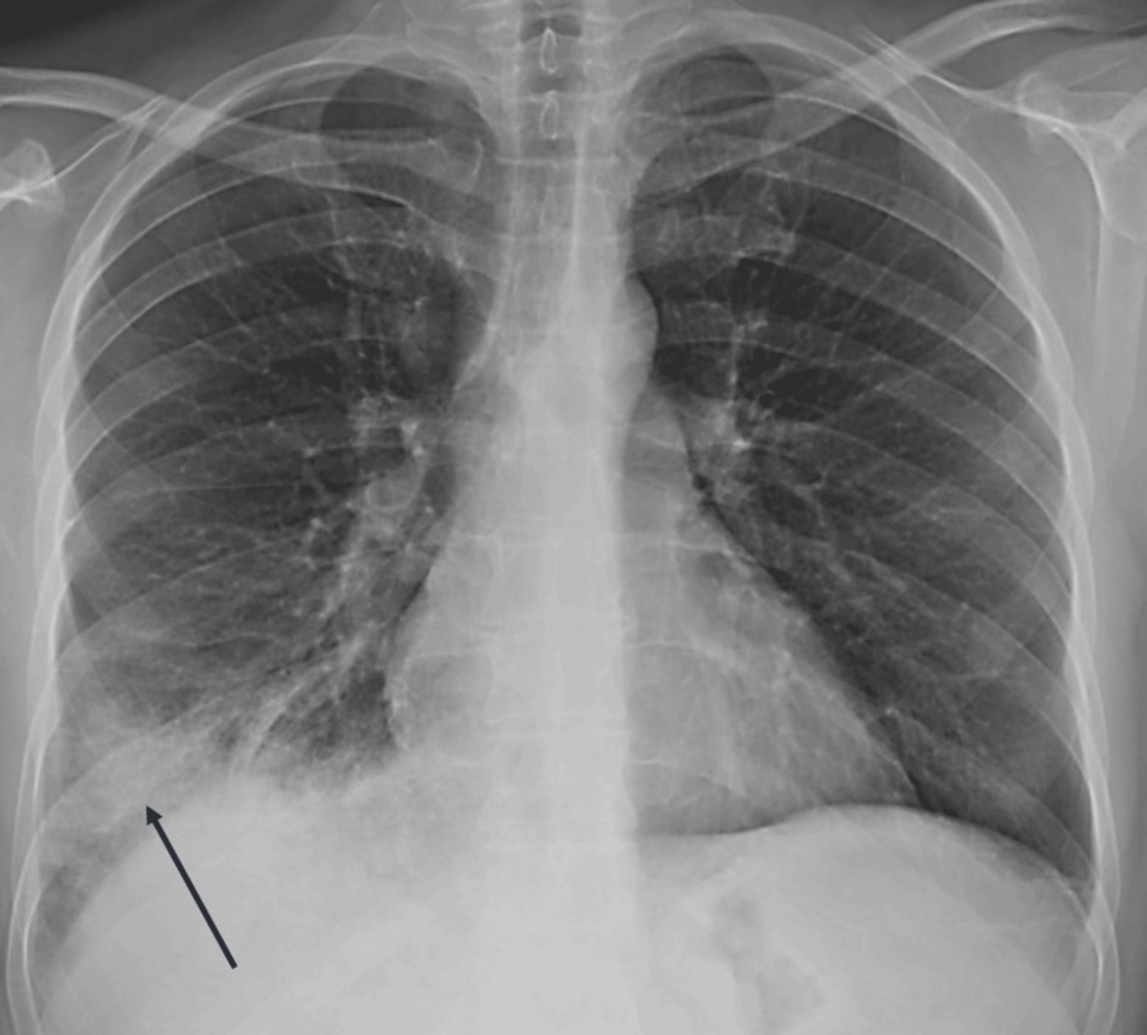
Chest X-ray on admission showing confluent alveolar infiltrates in the right lower lung field with air bronchograms (arrow), consistent with right lower lobe pneumonia.
